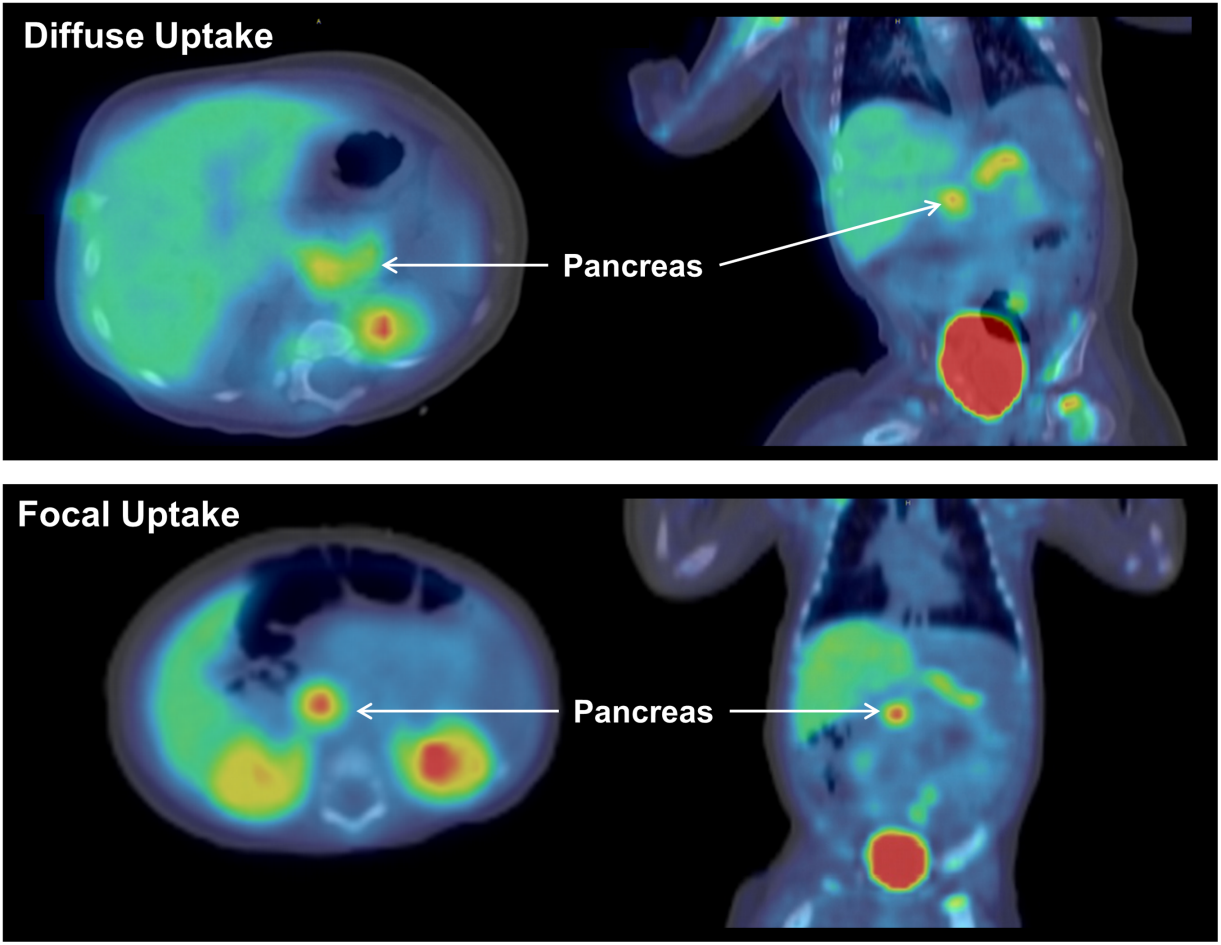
^18^F-fluoro-L-DOPA PET/CT scan showing the focal and diffuse uptake pattern in the pancreas axial (left) and coronal (right) view of ^18^F-Fluoro-L-DOPA scans from two representative subjects with hyperinsulinism. The top row shows a diffuse uptake of ^18^F-Fluoro-L-DOPA in the pancreas and the bottom row shows an intense focal accumulation of ^18^F-Fluoro-L-DOPA in the pancreas. Both the images were acquired at ~20 min post ^18^F-Fluoro-L-DOPA injection.
